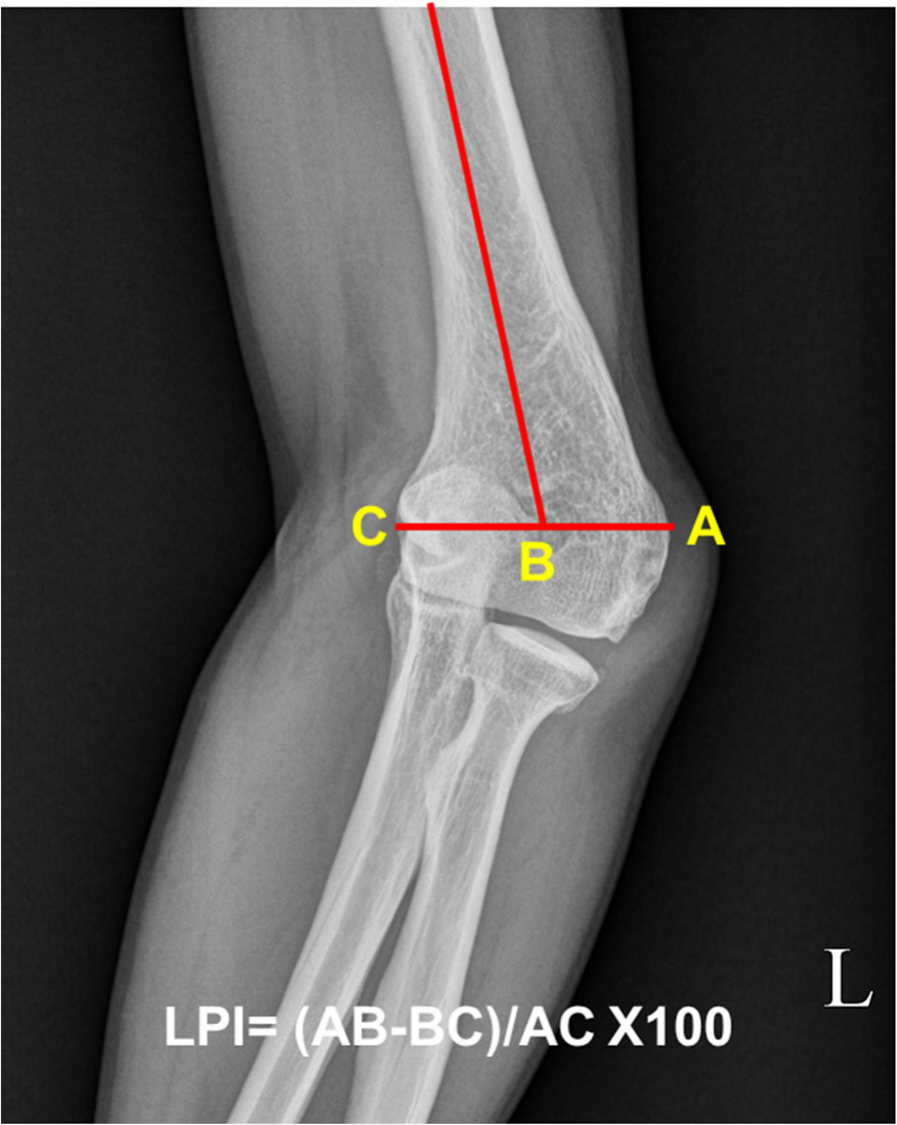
The lateral prominence index (%) is measured on preoperative and postoperative radiographs using the following formula: (AB-BC)/AC × 100. B, cross-link between a line connecting the lateral prominence; A, medial prominence; and C, longitudinal medhumeral axis [16]. This index is usually negative for normal elbows
